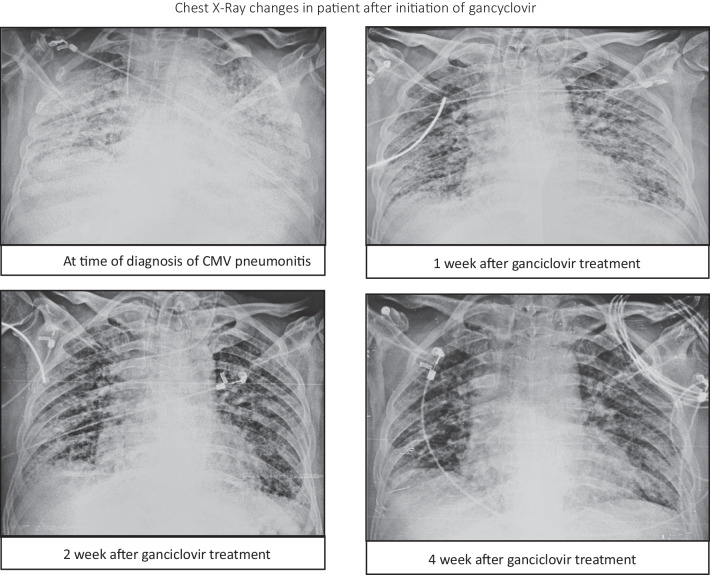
Chest X-ray changes in patient after initiation of ganciclovir
